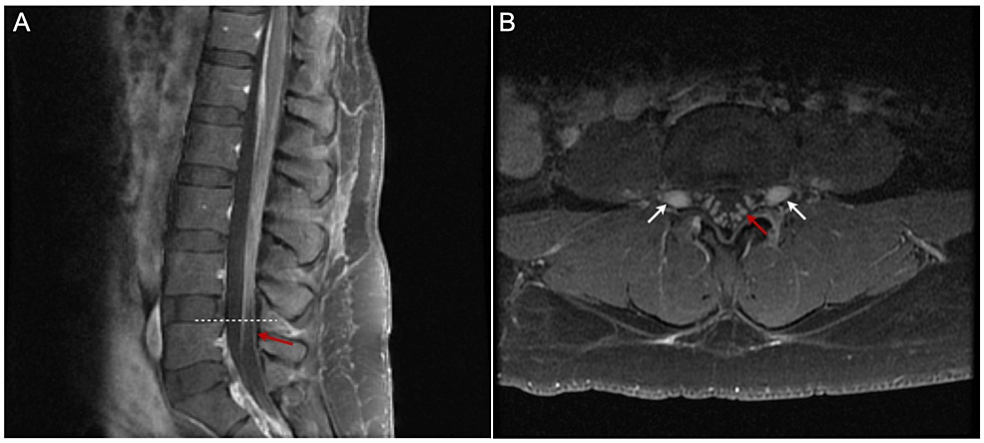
Lumbar spine magnetic resonance imaging (MRI) of a 41-year-old female with postpartum Guillain-Barré syndrome. Post-contrast fat-suppressed sagittal T1-weighted MRI (A) and axial T1-weighted MRI (B) showed mild smooth thickening and prominent enhancement of the cauda equina (red arrows in A and B) and exiting nerve roots (white arrows in B). The level of Figure 1B is shown as the dotted line in Figure 1A.
